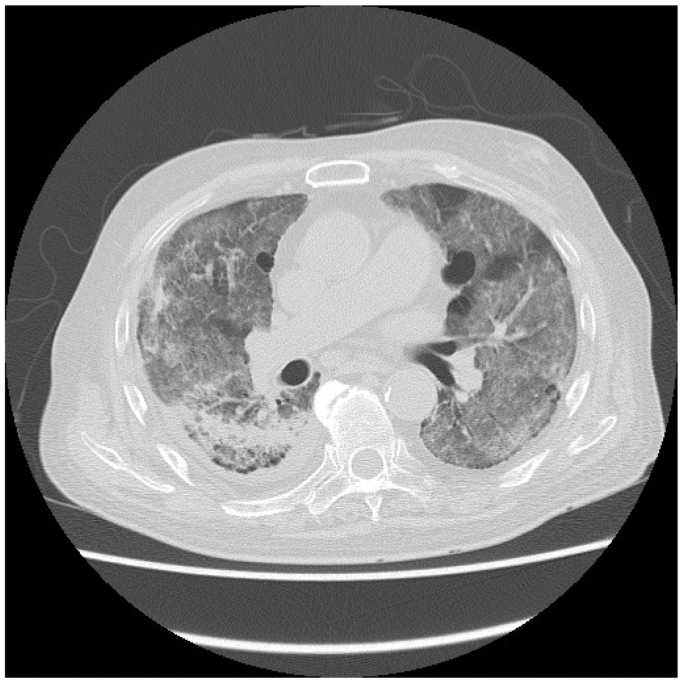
Chest computed tomography (CT) showing bilateral ground-glass opacities, predominantly in the peripheral and lower lung zones.
